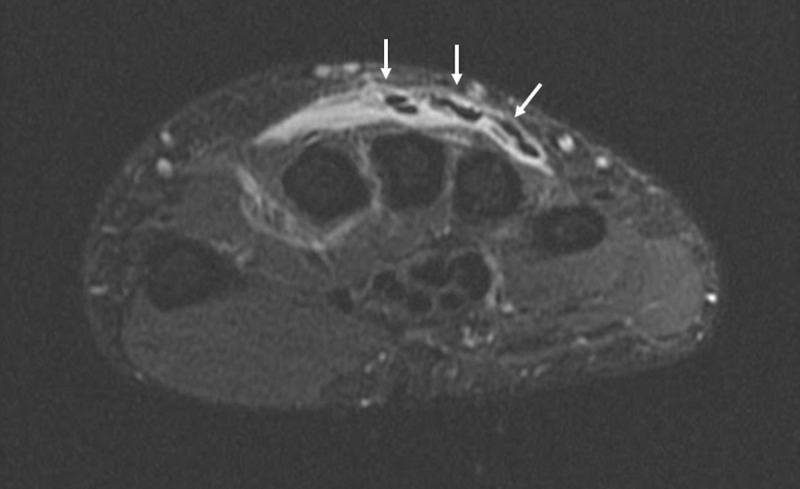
MRI of the right hand with contrast, axial view. Inflammatory edematous changes surrounding the extensor tendons of the second, third, and fourth compartments (white arrows), and to a lesser extent the fifth and sixth extensor compartments. The second, third, and fourth extensor compartments with mild synovial enhancement involving the tendon sheaths.
